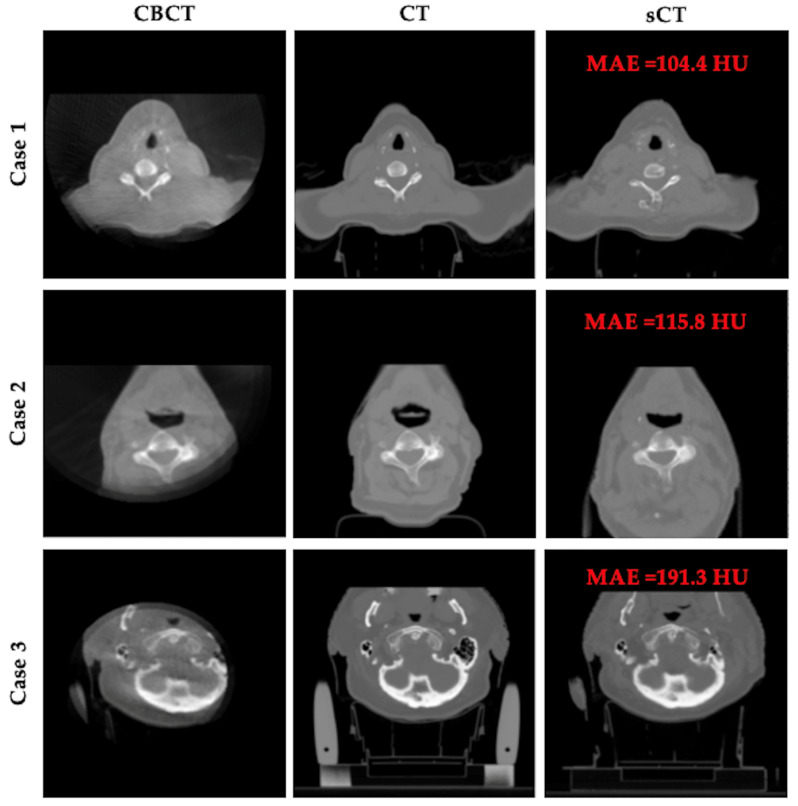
Representative failure cases. Each row shows CBCT, planning CT, and sCT results for a patient.
